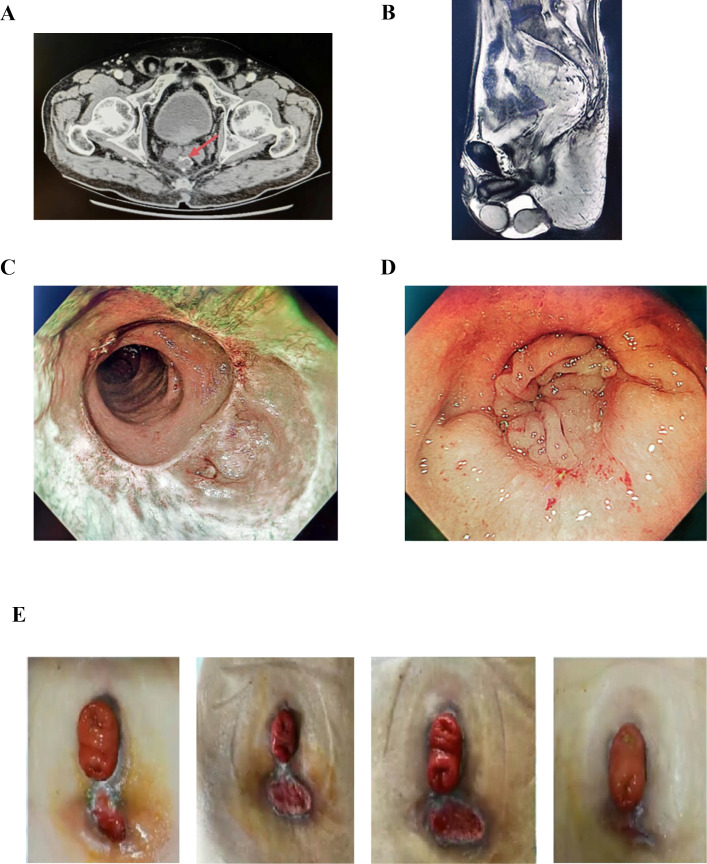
Preoperative assessment of anastomotic and stoma in patients who have undergone EC or RC. (A–D). Preoperative assessment of anastomotic integrity in patients who have undergone early 3 weeks ileostomy closure. (A) CT demonstrates that the arrow indicates that the anastomotic site and anastomotic staples are visible, with smooth margins and no significant abnormal enhancement observed. (B). MRI demonstrates that the anastomotic site has healed well, and the distal bowel is patent. (C, D). electronic colonoscopy demonstrates that the mucosa at the anastomotic site is smooth, with no ulcers or masses observed; anastomotic staples are visible; the intestinal lumen is patent; (E): Peristomal pyoderma gangrenosum and its treatment.
